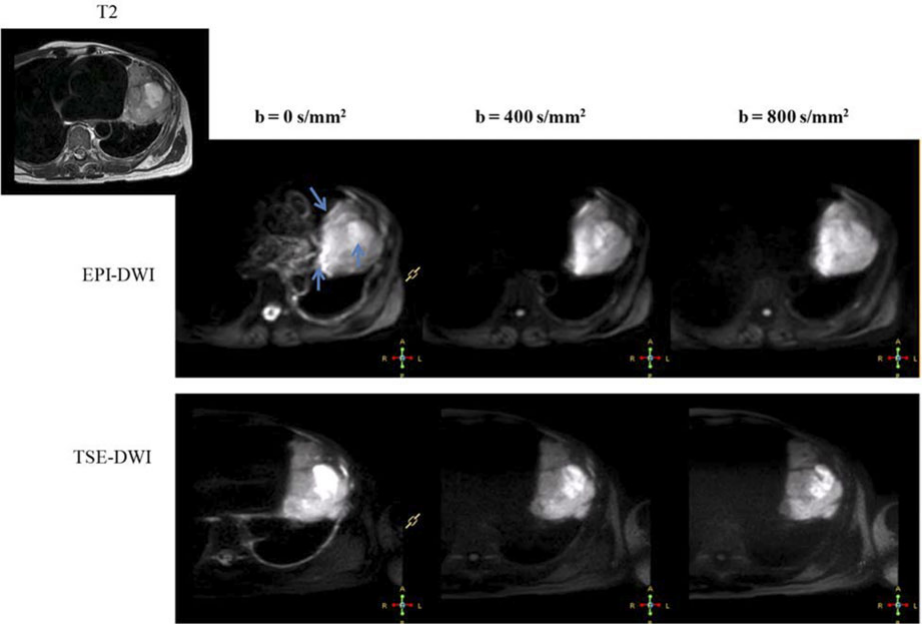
Diffusion‐weighted echo‐planar imaging (EPI‐DWI) and diffusion‐weighted turbo spin‐echo (TSE‐DWI) images of an example patient (patient 10). Images corresponding to b = 0, 400, and 800 s/mm^2^ are shown. Top left image shows the T2w magnetic resonance image of the tumor location. Blue arrows show the regions of susceptibility artifacts of EPI‐DWI.
